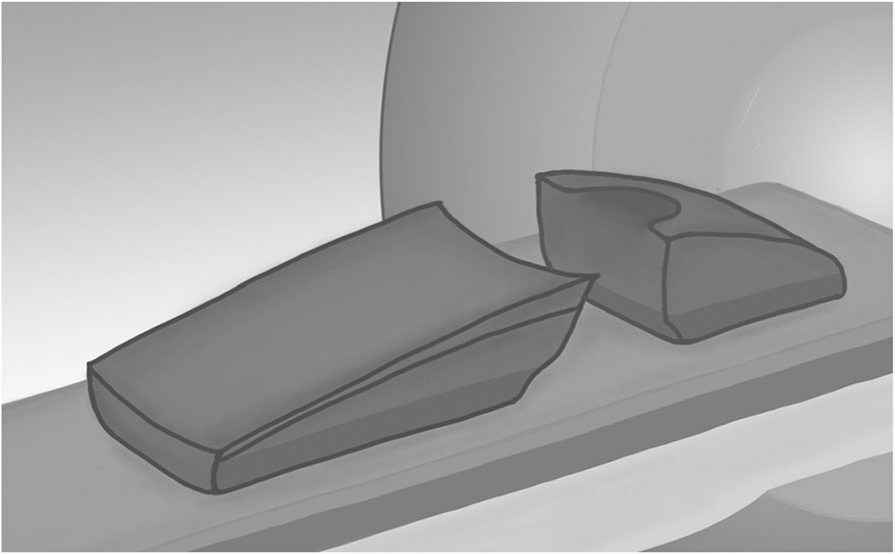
Sketch of the positioning aid for patients in prone position with both breasts hanging freely in photon-counting computed tomography like in breast magnetic resonance imaging
